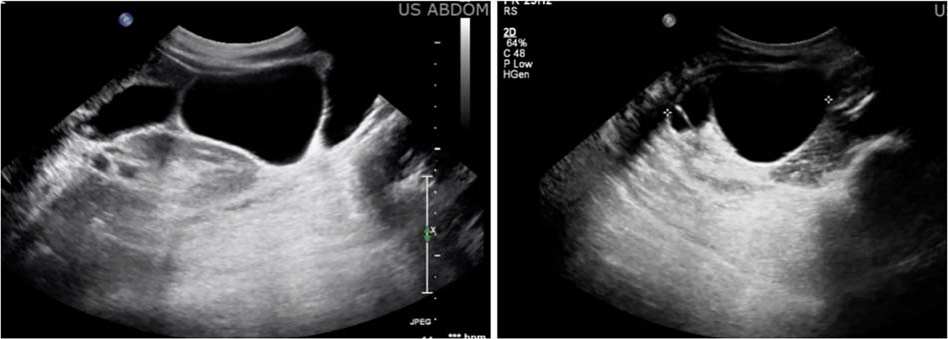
Ultrasound imaging revealing a multiseptated cystic mass measuring 4.4 × 7.7 × 9.3 cm, suspicious for exophytic complex hepatic cyst.
